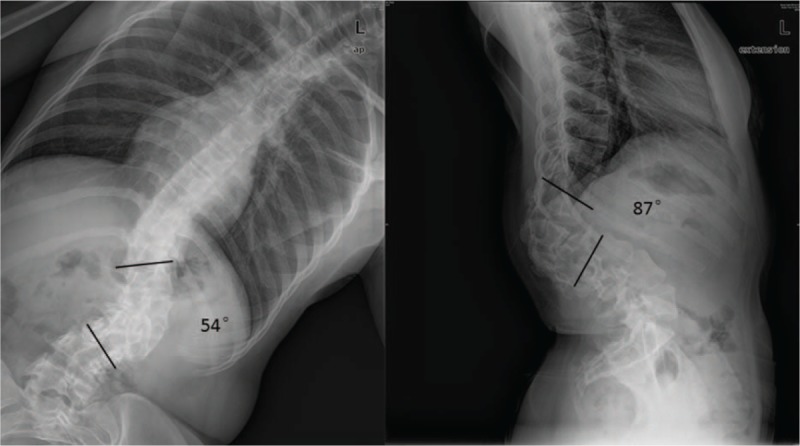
Flexion/extension X-ray.
